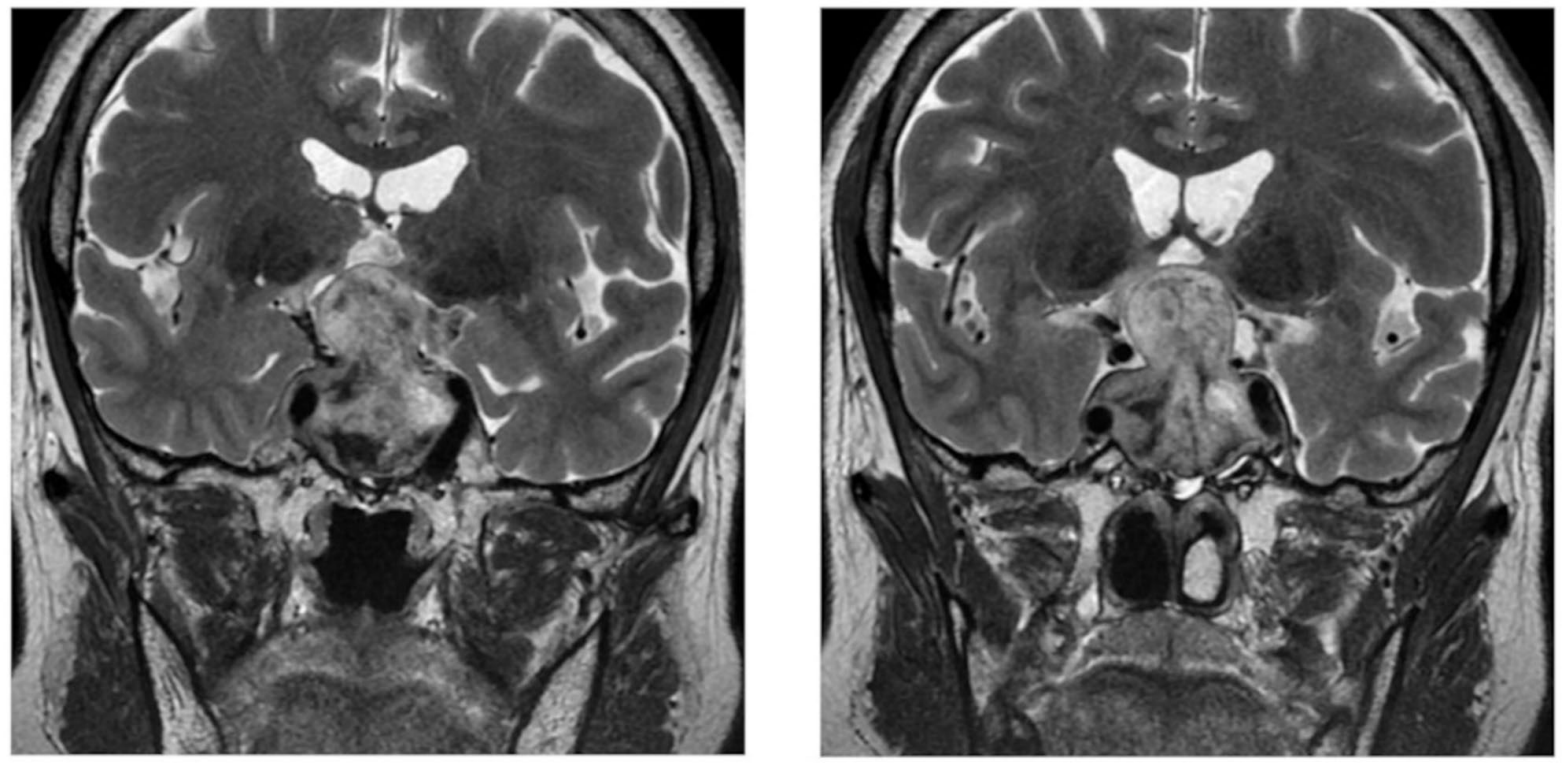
Contrast-enhanced magnetic resonance imaging on day 8 after onset of pituitary apoplexy. Contrast-enhanced magnetic resonance images showing a 33 × 27 × 43 mm mass extending from the intrasellar to suprasellar region. The lesion contained heterogeneous high-signal intensity on T2-weighted images, suggesting hemorrhage and necrosis
